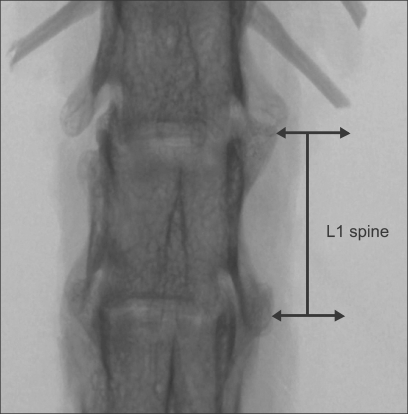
X-ray transmission image.
